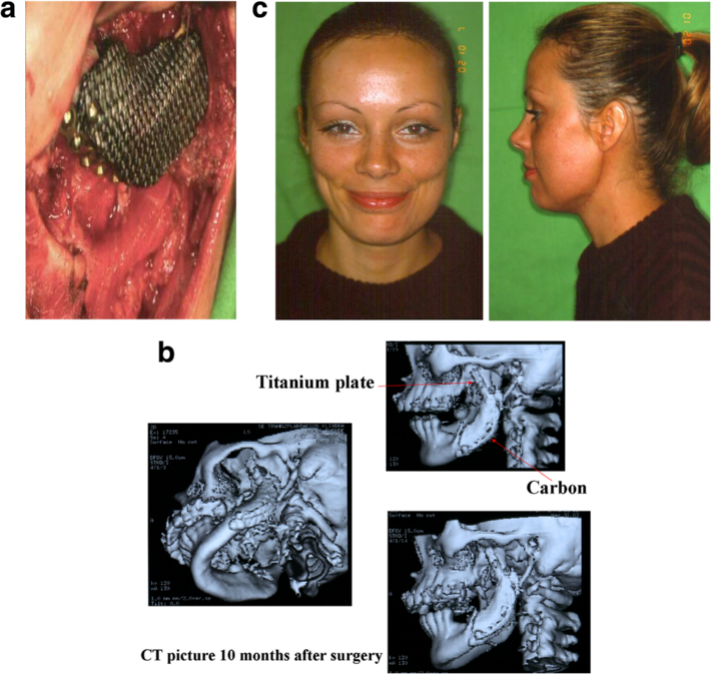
A 2-mm-thick net. a Resection of the mandible (synovial sarcoma) 2-mm-thick carbon/carbon net is screwed to the external surface of the mandible, follows the curvature of the bone. b CT picture after surgery. c 3 years after surgery
